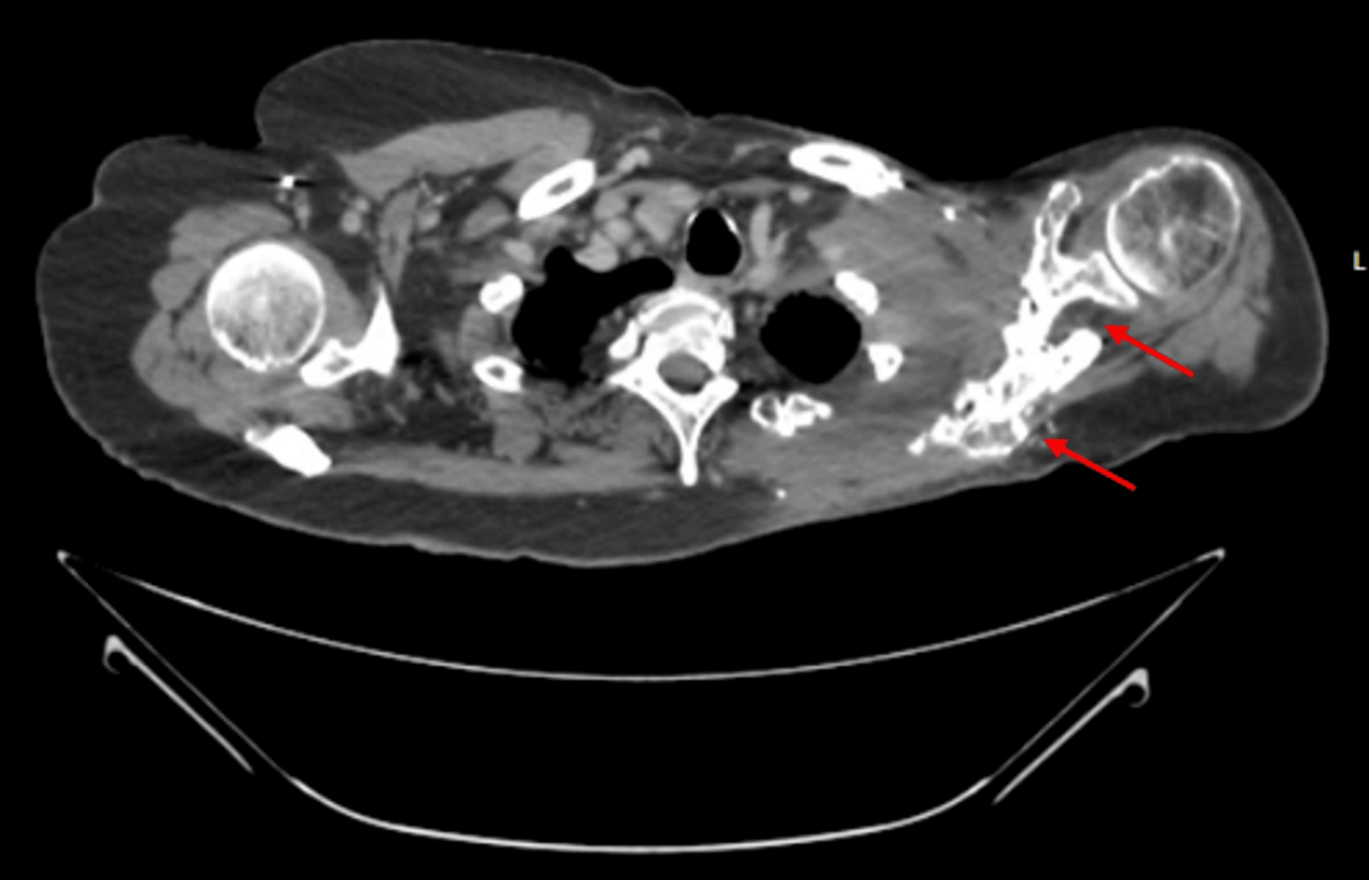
Axial computed tomography image of patient with infiltrate and sclerosis of left scapula.
